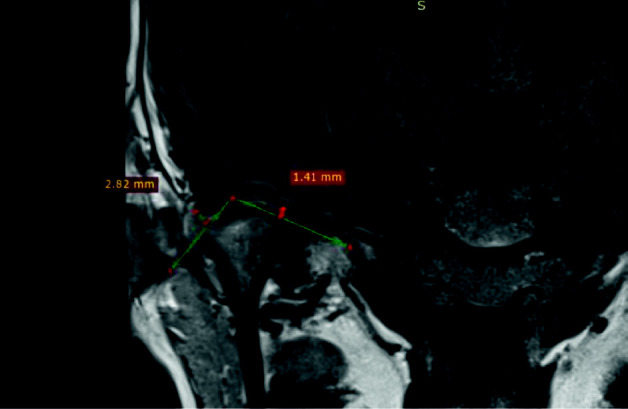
Measuring the TMJ space in MRI coronal view (medial pole = 1.41 mm and lateral pole = 2.82 mm).
